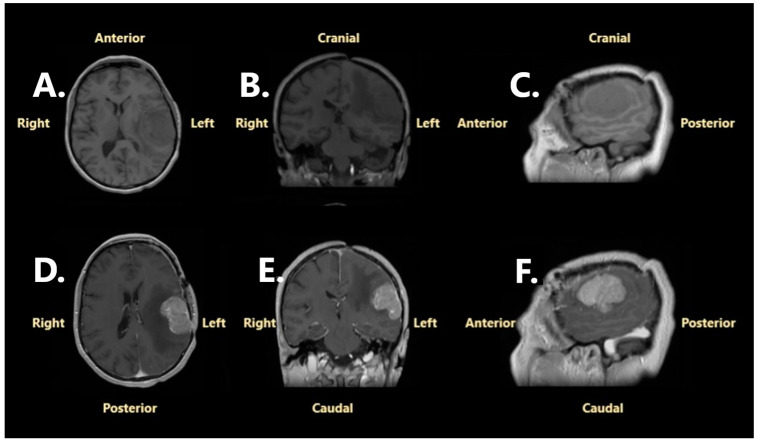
Brain MRI performed in August 2024; (A)—native T1-weighted MPR sequence, axial projection; (B)—native T1-weighted MPR sequence, coronal projection; (C)—native T1-weighted MPR sequence, sagittal projection; (D)—T2-weighted FLAIR FS sequence, axial projection; (E)—T2-weighted FLAIR FS sequence, coronal projection; (F)—3D T2 FLAIR FS sequence, sagittal projection.
